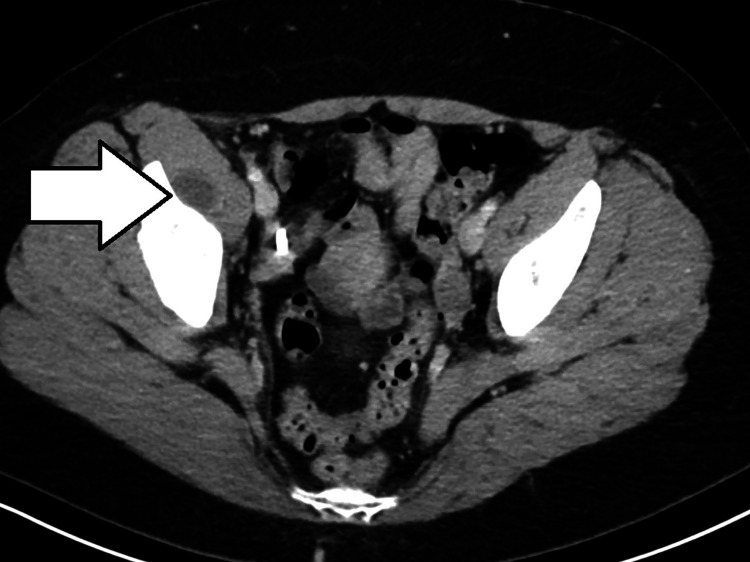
CT with contrast (transverse image) with right-sided iliopsoas bursitis. CT: computed tomography.
